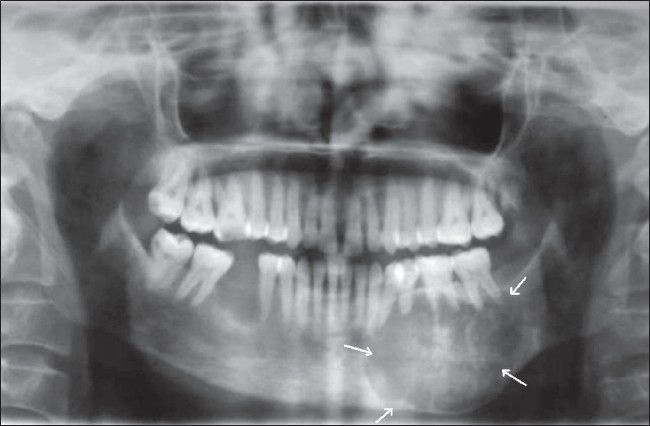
Orthopantomogram (OPG) shows a well-defined multilocular radiolucency (arrows) extending from teeth 34 to 37
